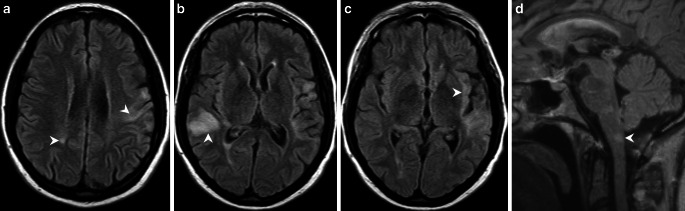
Axial fluid-attenuated inversion recovery (FLAIR) images show multiple hyperintense cortico-subcortical and white matter lesions. These lesions are located in the left precentral gyrus and the right-sided parietal white matter (a, *arrowheads*), the right superior temporal gyrus and angular gyrus (b, *arrowhead*), the left-sided insular cortex and subcortical areas (c, *arrowhead*), as well as dorsal parts of the medulla oblongata (d, *arrowhead*). The corpus callosum is not affected (d)
